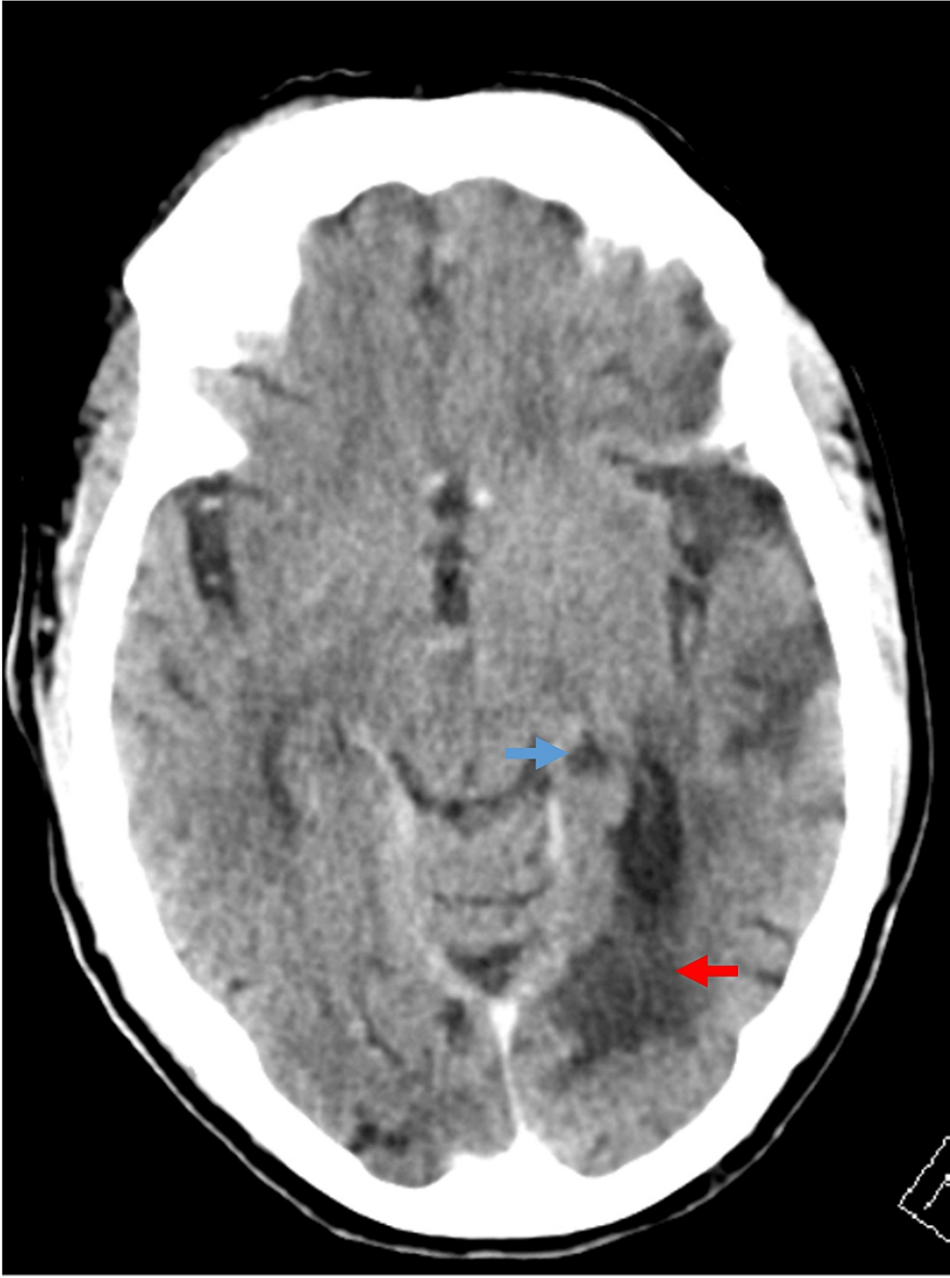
Case 2: Hypodensity in the medial temporal lobe (red arrow) and the splenium of the corpus callosum on a noncontrast CT head (blue arrow)
